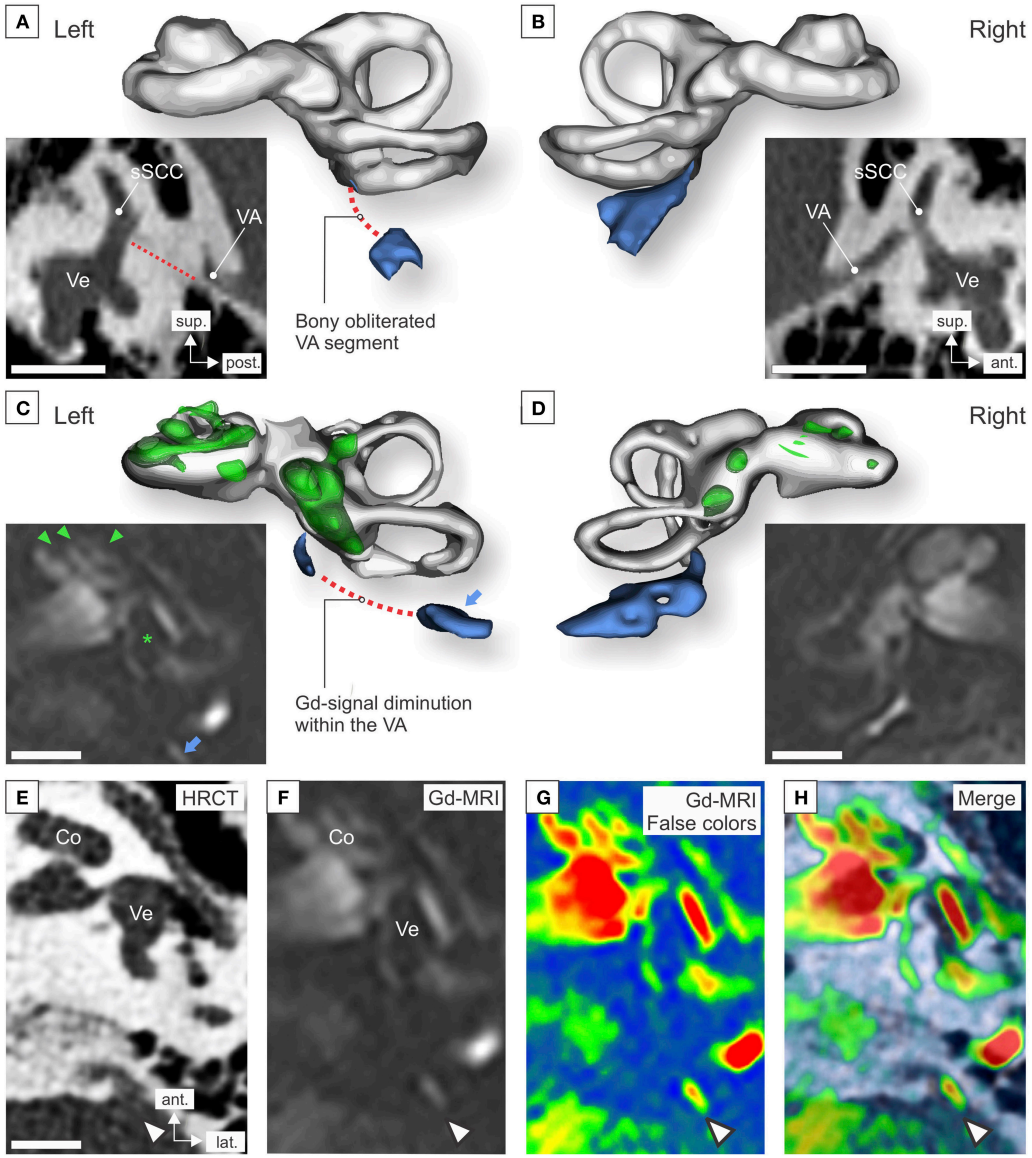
High-resolution CT (HRCT) imaging and gadolinium-enhanced MRI (Gd-MRI) of the inner ears was performed after the onset of Meniere's symptoms (HRCT, at age 44; Gd-MRI, at age 48). (A,B) Sagittal HRCT projections and 3D segmentations of the left (A) and right (B) labyrinth. (VA, vestibular aqueduct (blue in 3D segmentation); sSCC, superior semicircular canal; Ve, vestibule; red dotted line in (A), bony obliterated VA. (C,D) Axial Gd-MRI (3D inversion recovery sequence) and 3D segmentations of the left (C) and right (D) labyrinth. Signal voids in the cochlear turns (green arrowheads) and the saccule (green asterisk) indicate endolymphatic hydrops; blue arrow, signal originating from the extraosseous endolymphatic sac. 3D segmentations were performed using the 3D Slicer software [version 4.6.2, (12)]. (E–H) Fusion of axial HRCT (E) and Gd-MRI [F; false color-coded image in (G)] images of the left temporal bone in the corresponding axial plane (H). Arrowheads (E–H): T2 signal localized posterior to the operculum, corresponding to the localization of the distal (extraosseous) endolymphatic sac (D). (Co, cochlea; PSCC, posterior semicircular canal; VA, vestibular aqueduct; Ve, vestibule). Scale bars: 5 mm. The false color-coding image overlay was generated using Adobe Photoshop CC2017 (version 18.1.0, San Jose, CA, USA).
